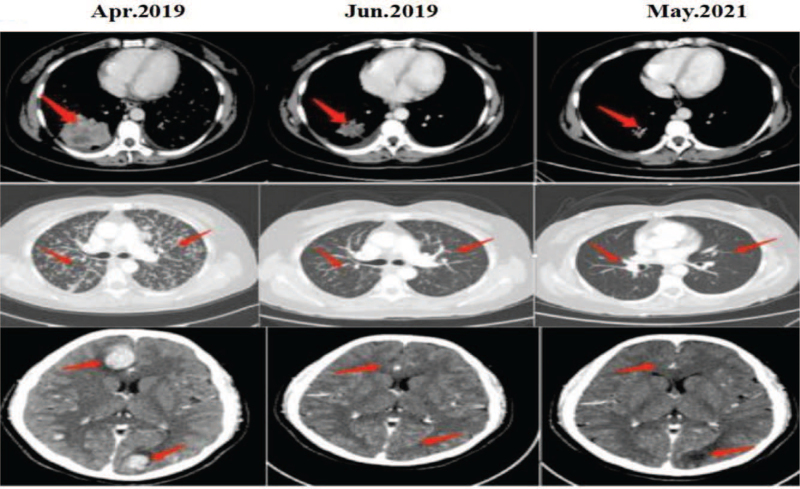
Radiographic response to treatment. April 2019: chest CT scan performed before alectinib treatment (March 10, 2020) showed a 6.7 × 5.4 cm irregular mass and multiple enlarged lymph nodes can be seen in both hilum, mediastinum and double clavicle, and the large one have a short diameter of about 1.2 cm; Multiple enhanced nodules and masses can be seen in bilateral brain parenchyma, with a diameter of about 2.7 cm and a low density edema zone around part of the brain. June 2019: CT performed after 45 days of alectinib treatment (April 27, 2019) demonstrated a reduced tumor volume (2.7 × 3.4 cm) and the nodules and masses in the bilateral brain parenchyma were not clearly displayed, and there were low-density edema areas around the brain. All were dramatically reduce compared with the previous. May 2021: the intrapulmonary lesion was significantly smaller than before; lamellar hypodensity with indistinct borders was seen in the right frontal and left occipital lobes. Efficacy evaluation: PR.
